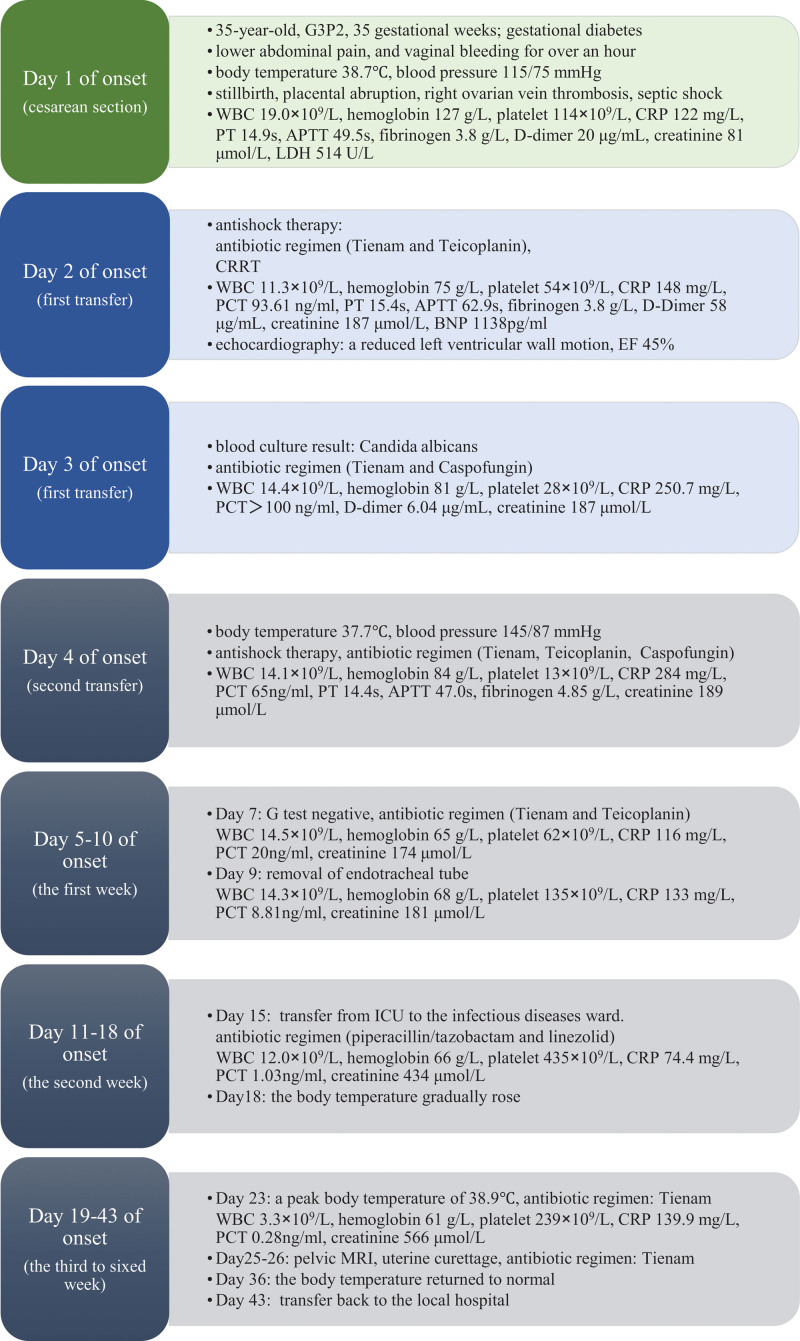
Timeline of events and findings. APTT = activated partial thromboplastin time, BNP = brain natriuretic peptide, CRP = C-reactive protein, CRRT = continuous renal replacement therapy, EF = ejection fraction, ICU = intensive care unit, LDH = lactate dehydrogenase, MRI = magnetic resonance imaging, PCT = procalcitonin, PT = prothrombin time, WBC = white blood cell count.
